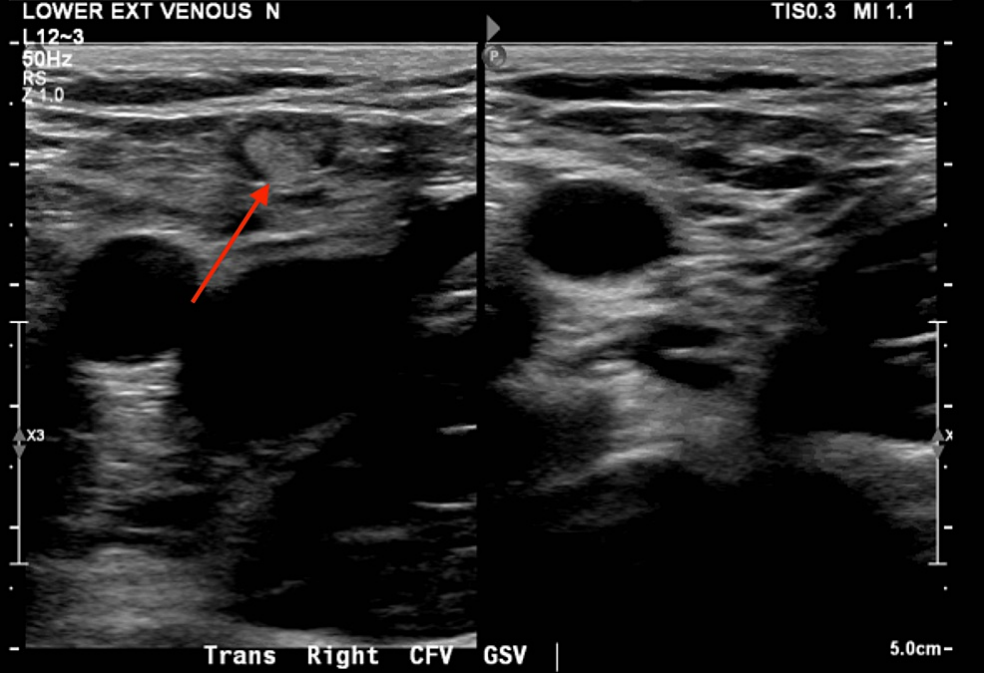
Bilateral lower extremity venous duplex ultrasound performed revealed occlusive thrombosis of the left distal posterior tibial vein.
